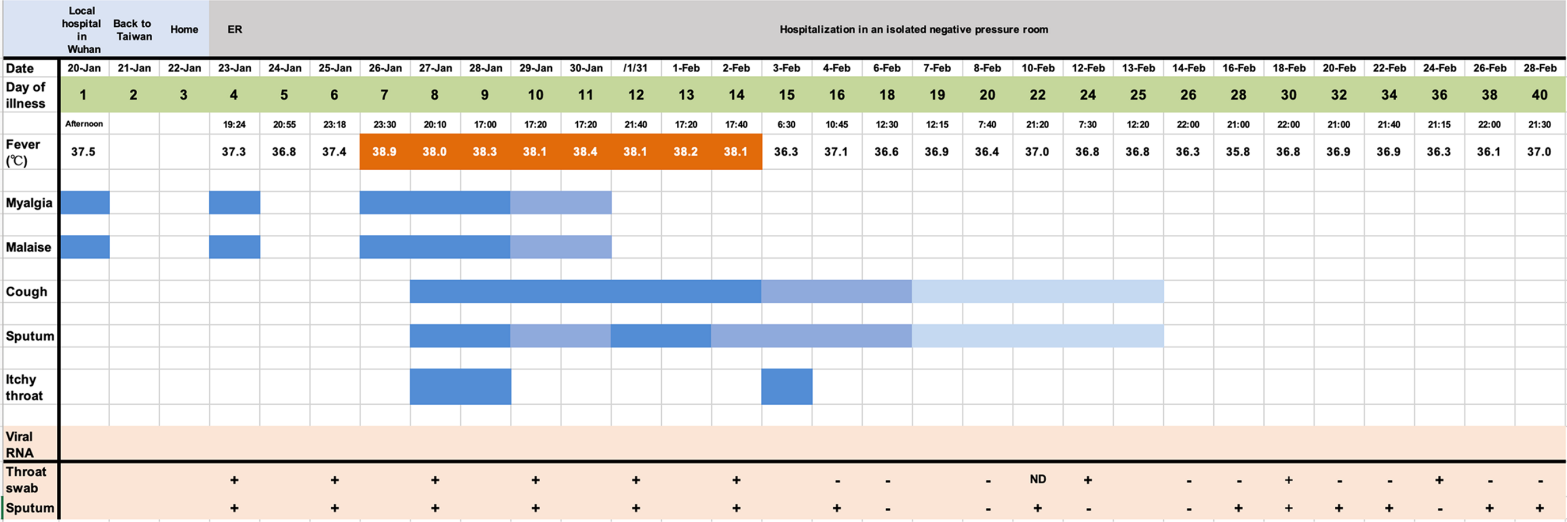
After admission, the patient’s white blood counts showed leucopenia (2.84×10^9^ l^−1^) on illness day 5 (Table 2) as reported [4, 5]. He received empiric antibiotic treatment with azithromycin (500 mg/day, illness day 4–8), then intravenous administration of ceftriaxone (1000 mg/12 h, illness day 8–16) and levofloxacin (750 mg/day, illness day 8–13) in association with supportive care. During the ensuing 6 days, he showed signs of mild fever, myalgia, malaise, and a peak fever (38.9 °C) on illness day 7. A more productive cough followed on the eighth day. He did not have nausea, vomiting, diarrhea or abdominal discomfort. Chest x-ray showed occasional infiltration with consolidation at bilateral lower lung field until illness day 23 (Figs S2–S10). The patient’s fever subsided after illness day 14. The late worsening of this illness to a high peak fever with pneumonia is consistent with recent reports [4, 5]. However, while rRT-PCR tests had been negative twice (on days 18 and 20 after illness) and clinical symptoms improved significantly, the virus could be detected again from day 22 (Table 1). The viral shedding was still evident after 14 days of recovering from respiratory symptoms (day 40). Currently the patient is still in hospital under observation. So far, no evidence of infection in family or the community has been found after the CDC followed up with the people he had contact with in Taiwan.
